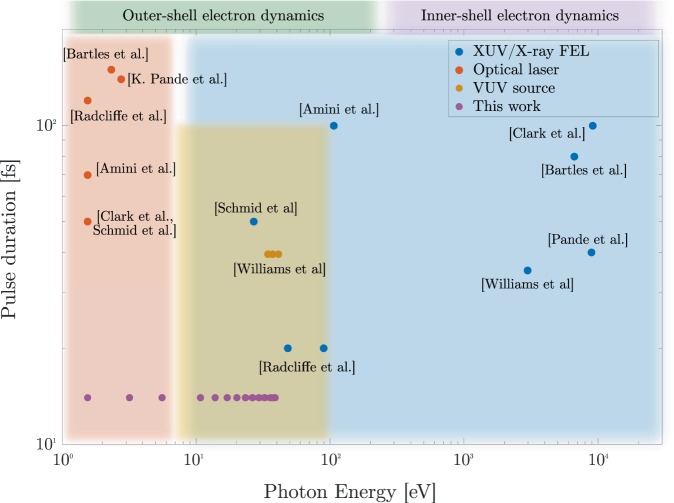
Pump-probe parameter ranges of selected experiments in which an external optical laser was used in combination with a XUV/X-ray FEL^35–41^. The pulse duration of pump and probe pulses is displayed together with the corresponding photon energy. Data labels and markers indicate references and instrument (blue: XUV/X-ray FELs, red: optical lasers including bulk-based frequency converted lasers, orange: VUV sources). The HHG-beamline described in this work is indicated in violet. Please note that the represented data are not intended to be exhaustive, but rather to give an overview on the available parameter spaces at FEL facilities.
